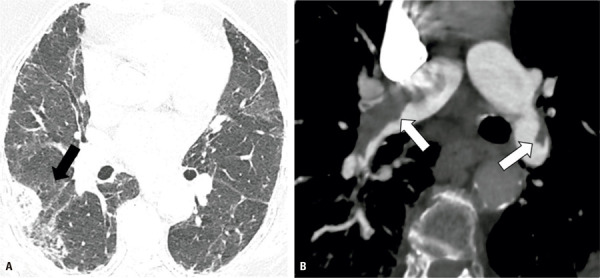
An 84-year-old woman with fibrosing interstitial lung disease of unknown aetiology and worsening dyspnoea. (A) Axial chest computed tomography image showing a new peripheral consolidation with a reversed halo morphology, suspicious of pulmonary infarction. (B) Computed tomography pulmonary angiography obtained subsequently, showing extensive filling defects in the main pulmonary arteries
